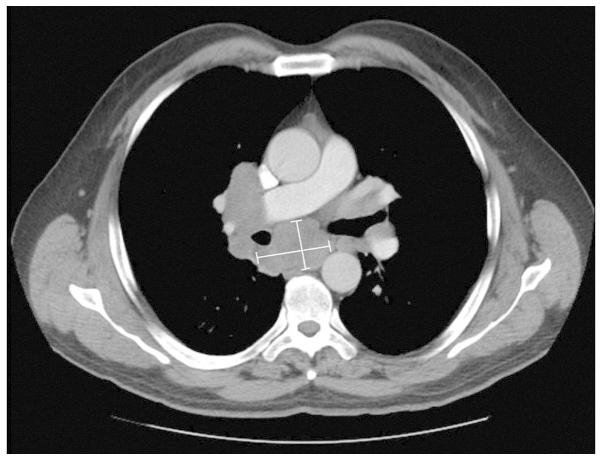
Computed tomography scan of the chest showing the large subcarinal and precarinal lymph nodes. White lines present the largest dimensions of the subcarinal lymph node observed (5.2×3.5 cm).
